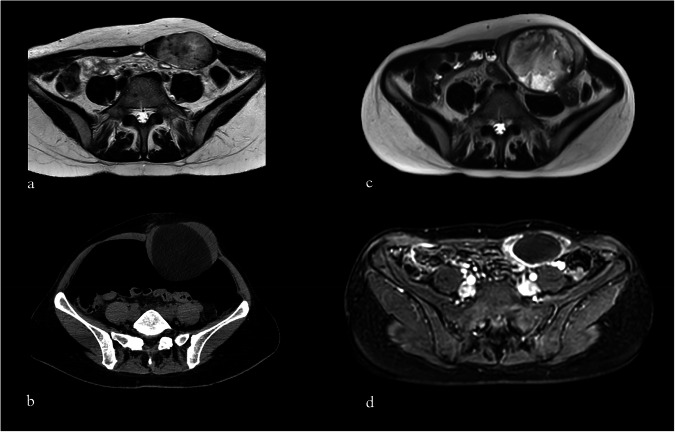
Partial response according to M-RECIST. a T2-weighted images at baseline; (b) intraprocedural CT imaging; (c) T2-weighted and (d) contrast-enhanced T1-weighted images after PC. Follow-up imaging (c, d) documented extensive colliquation of the lesion with persistence of peripheral soft tissue with intermediate T2 signal and late contrast enhancement, consistent with residual disease. The case was classified as SD according to RECIST 1.1 and partial response (PR) according to M-RECIST. CT imaging at the end of the procedure (b) already demonstrated undertreatment of the peripheral zone of the tumor
